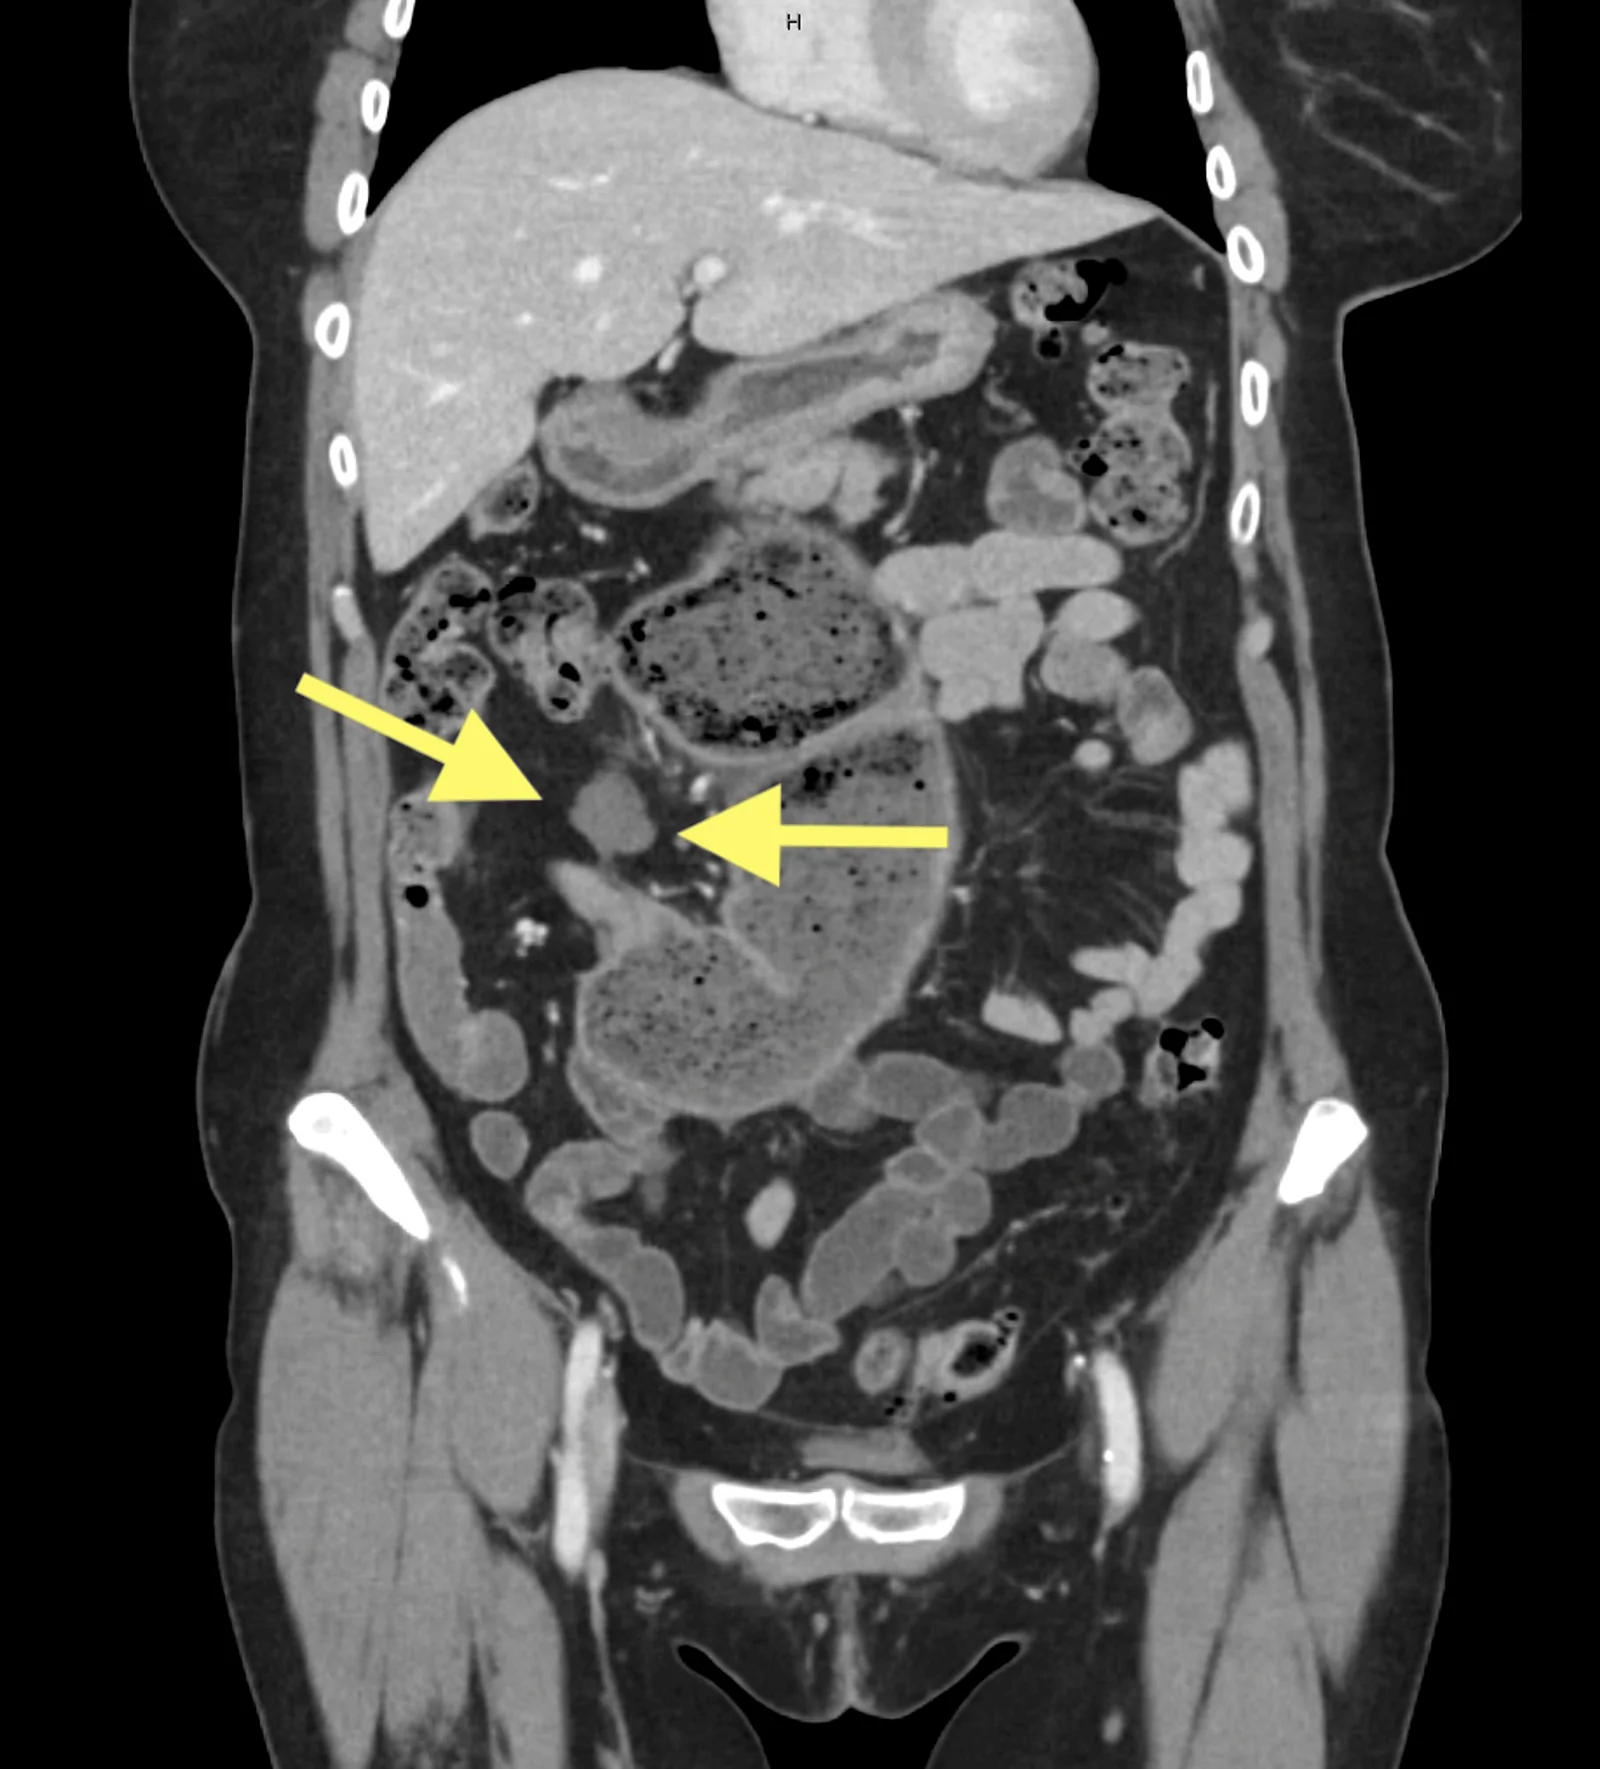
Coronal CT image showing marked dilation of the cecum and a transition point raising concern for cecal volvulus. Coronal CT image demonstrating marked dilation of the cecum extending superiorly and medially into the midabdomen. The yellow arrows indicate the site of torsion, with swirling of the mesenteric vessels (whirl sign), near the ileocecal transition zone, resulting in mechanical large bowel obstruction.
